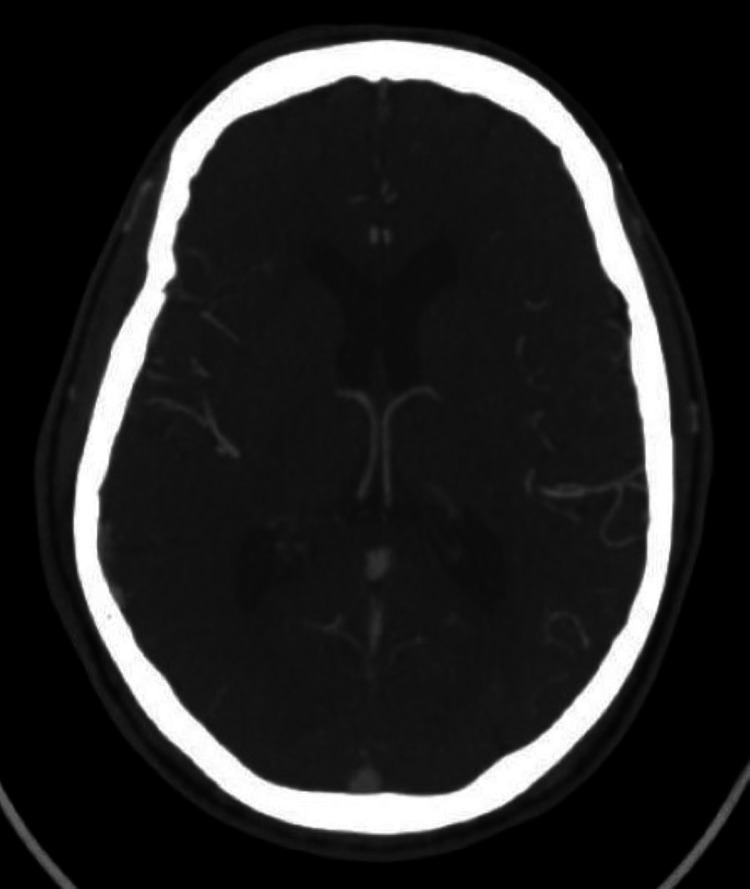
Computed tomography angiography of the head revealing chronic white matter microangiopathy with no arterial occlusion, obstructive stenosis, or aneurysm.
